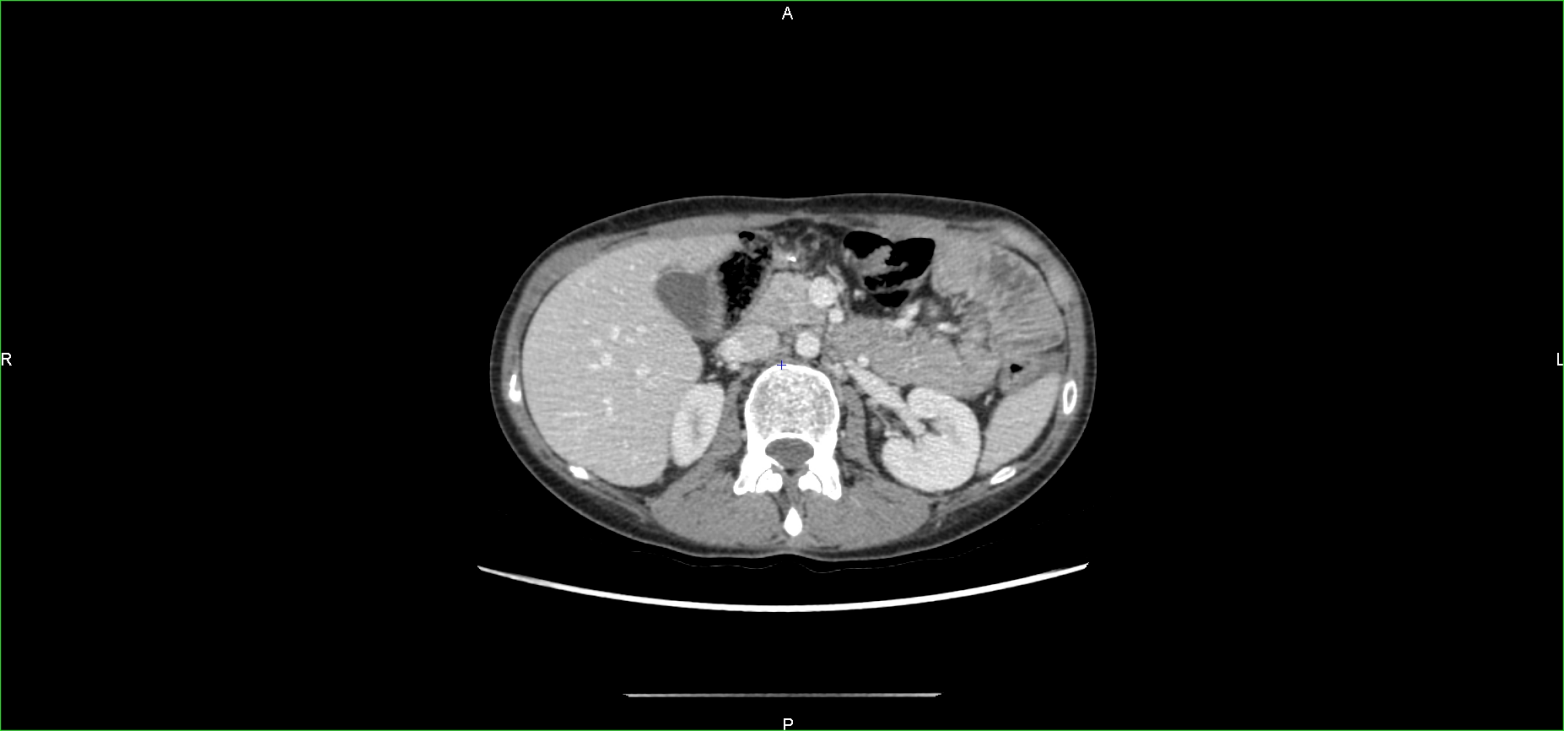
CT scan after combined treatment with chemotherapy and immunotherapy.
